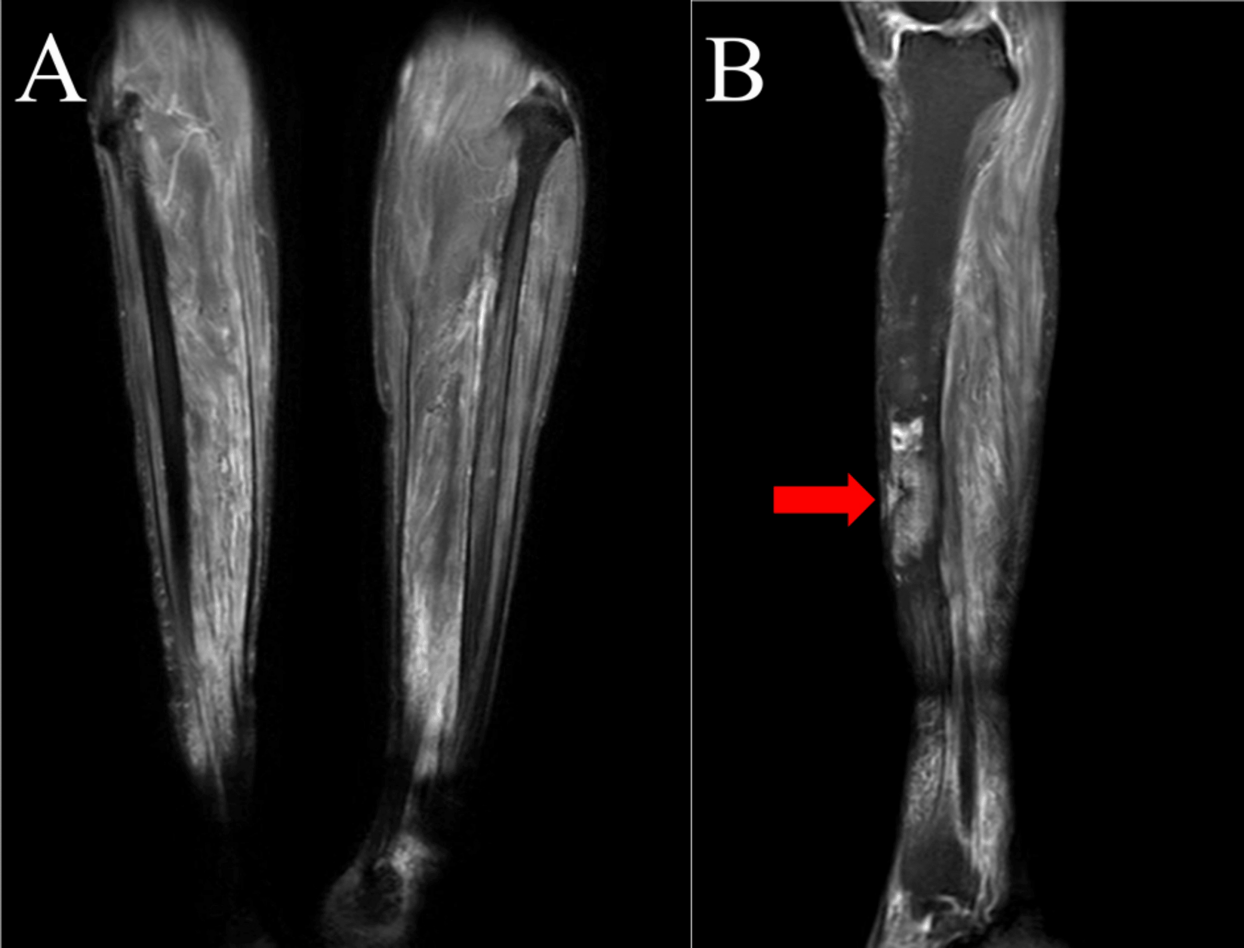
Two-month postoperative short-tau inversion-recovery (STIR) MRI findings (A) Coronal view shows resolution of preoperative signal abnormalities, consistent with bone healing; (B) Axial view confirms absence of cortical disruption or active inflammation (red arrowhead).
